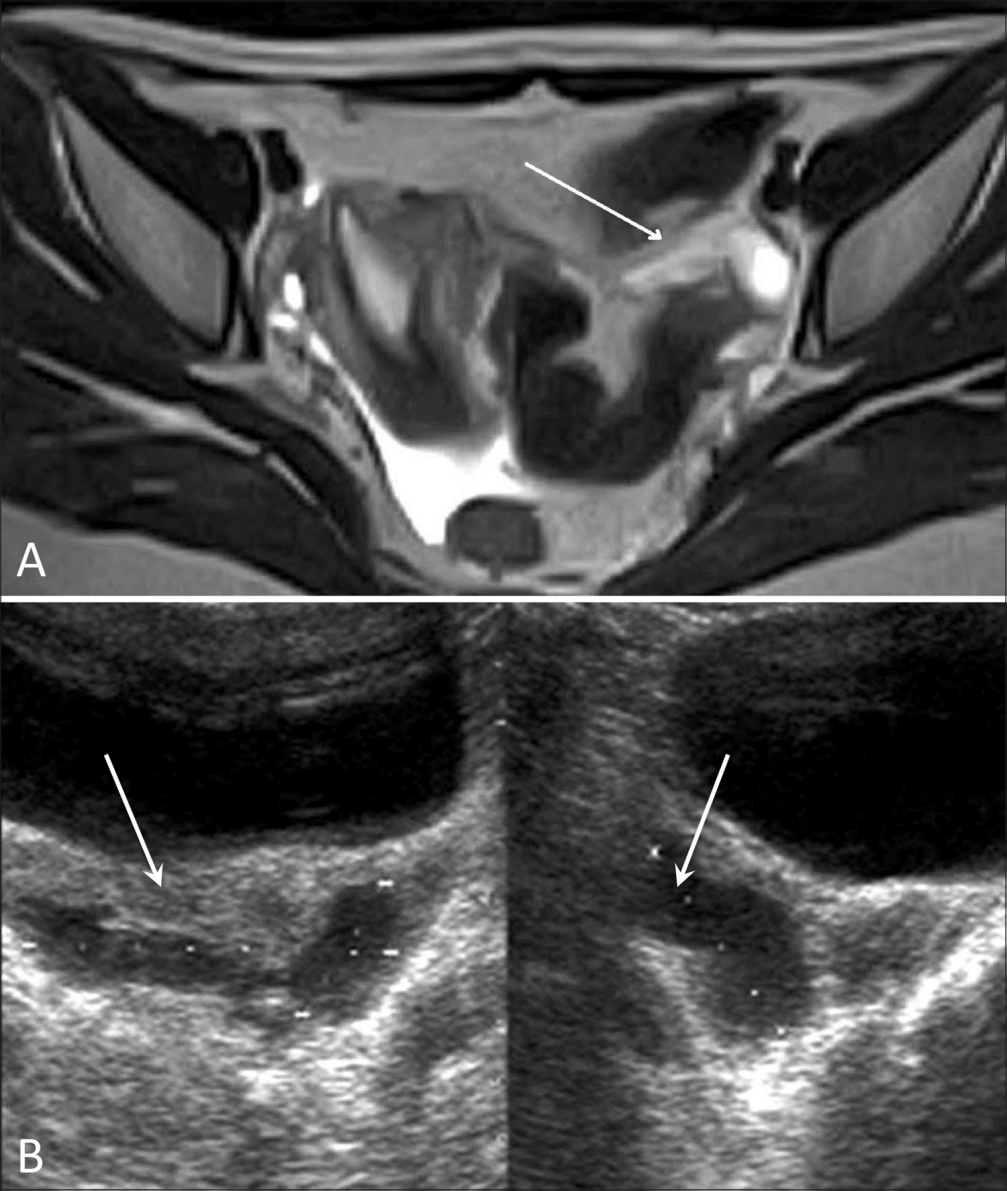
Pelvic inflammatory disease. Axial T2W MRI (A) and transabdominal USG (B) images show pelvic inflammatory disease with a digested/damaged left tube and mild collection in POD (white arrows)
